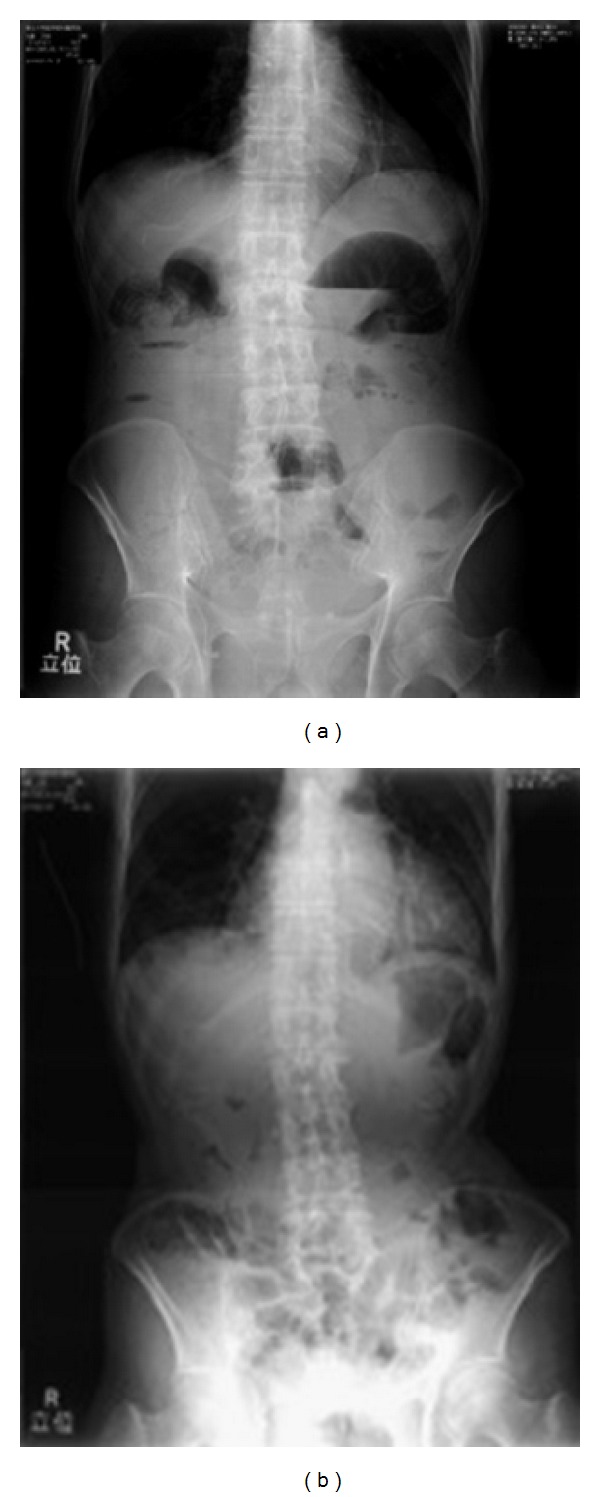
Abdominal X-ray radiographs of a 95-year-old paralytic ileus patient. (a) Radiograph taken at the time of hospital admission, (b) radiograph taken 18 days after moxibustion therapy.
